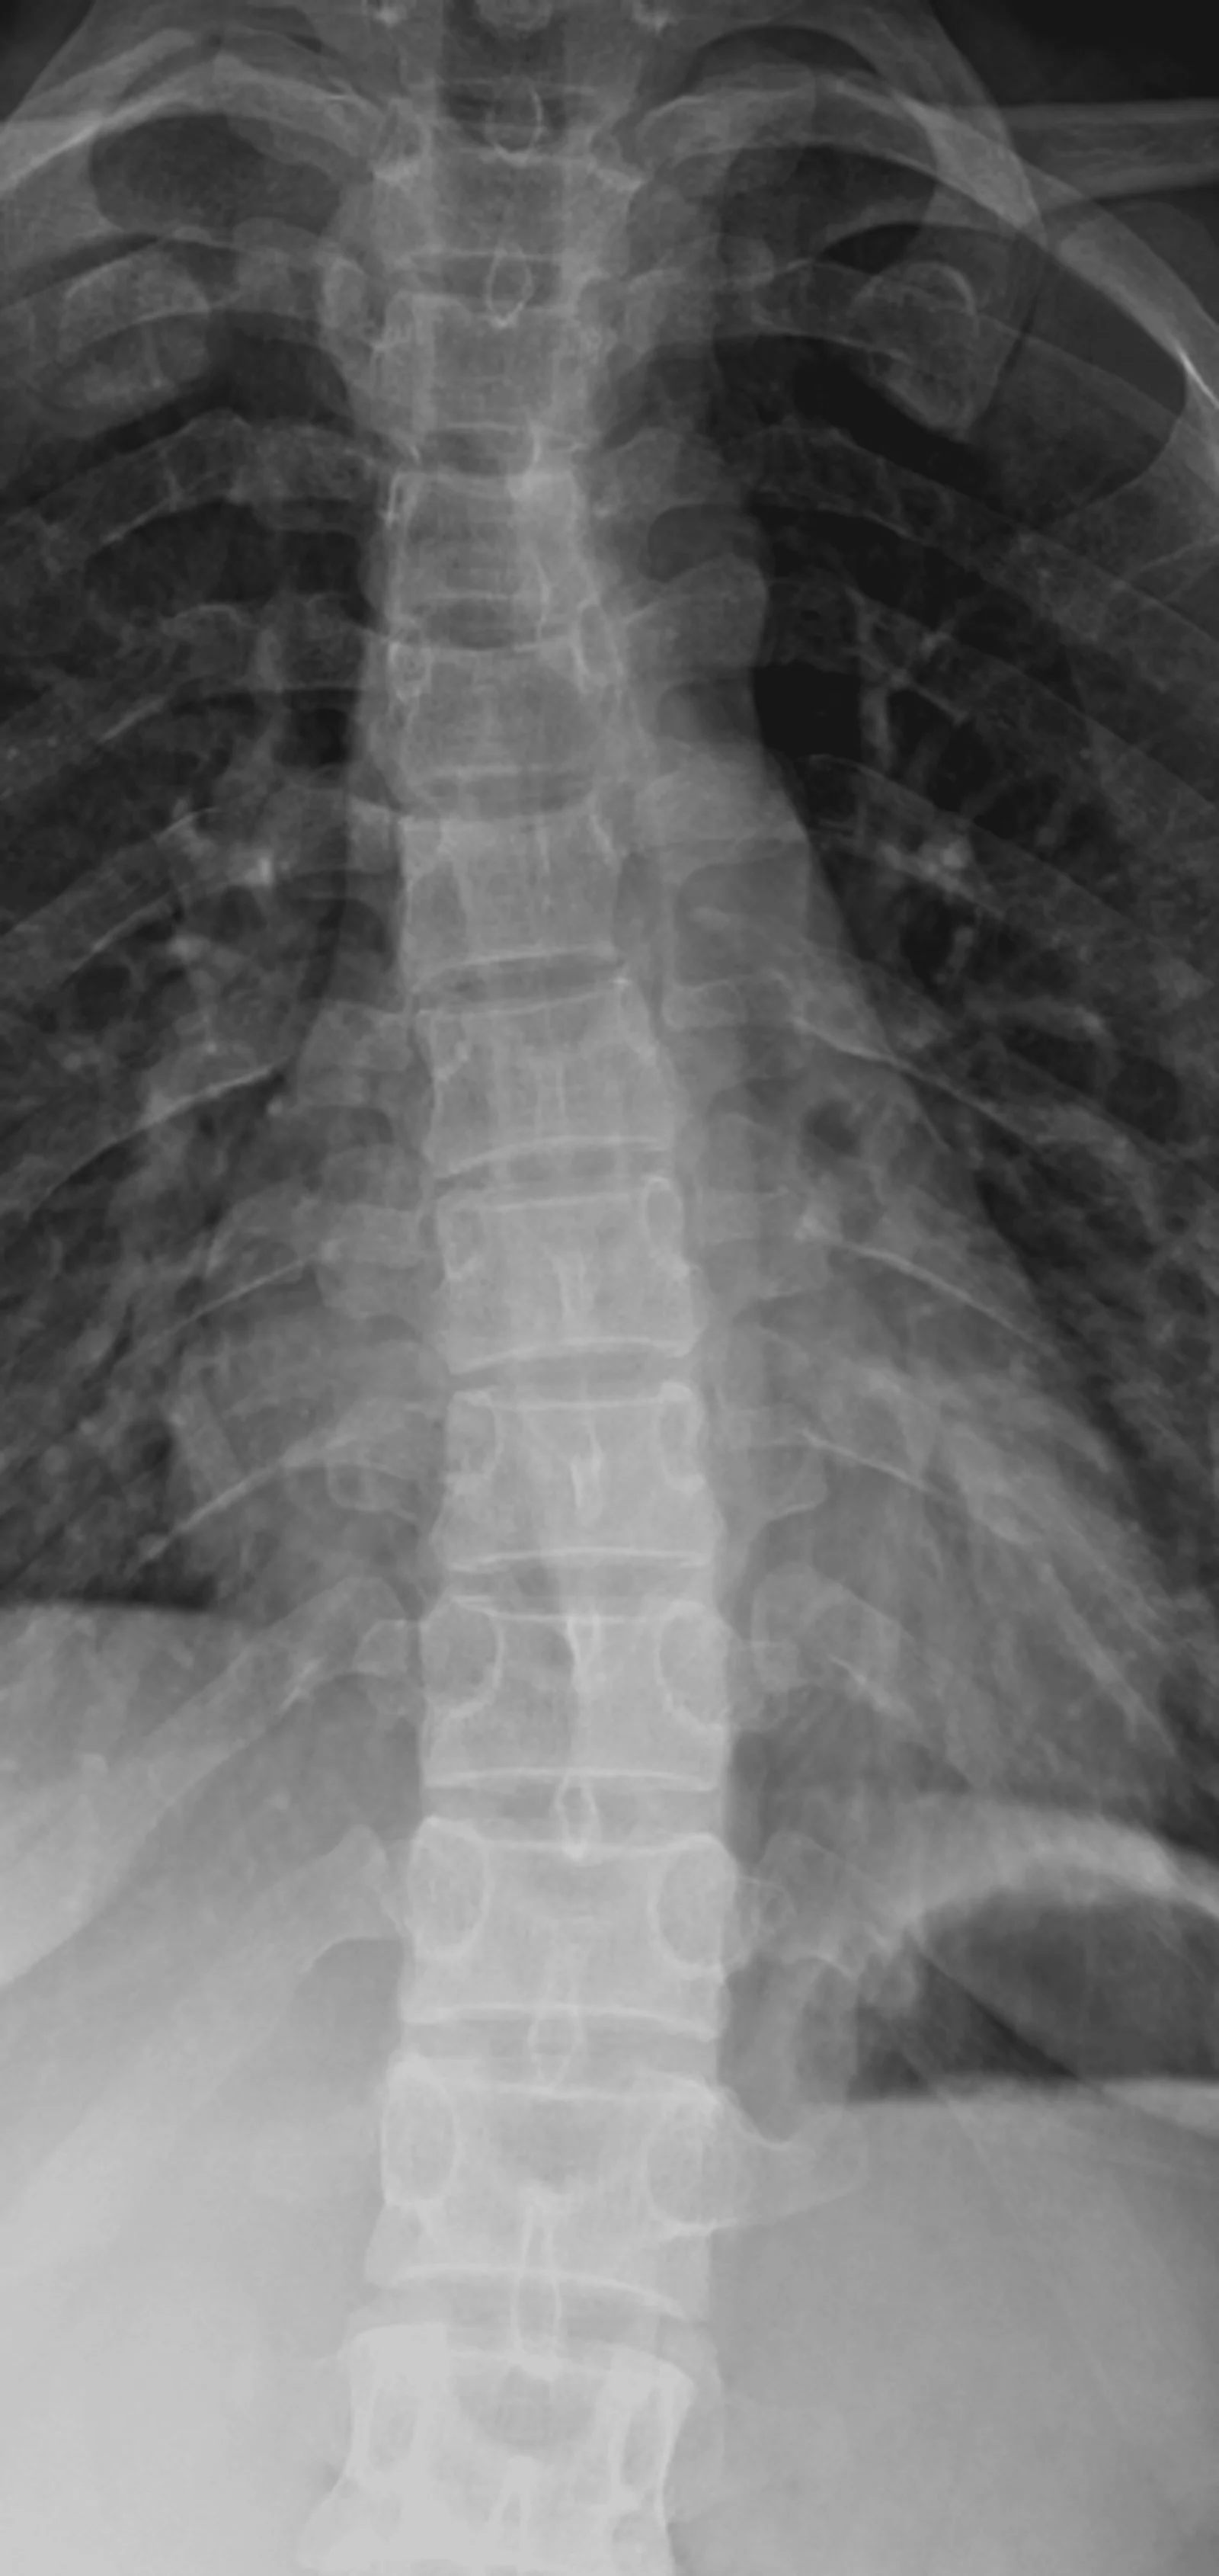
Thoracic radiograph demonstrating scoliosis.
